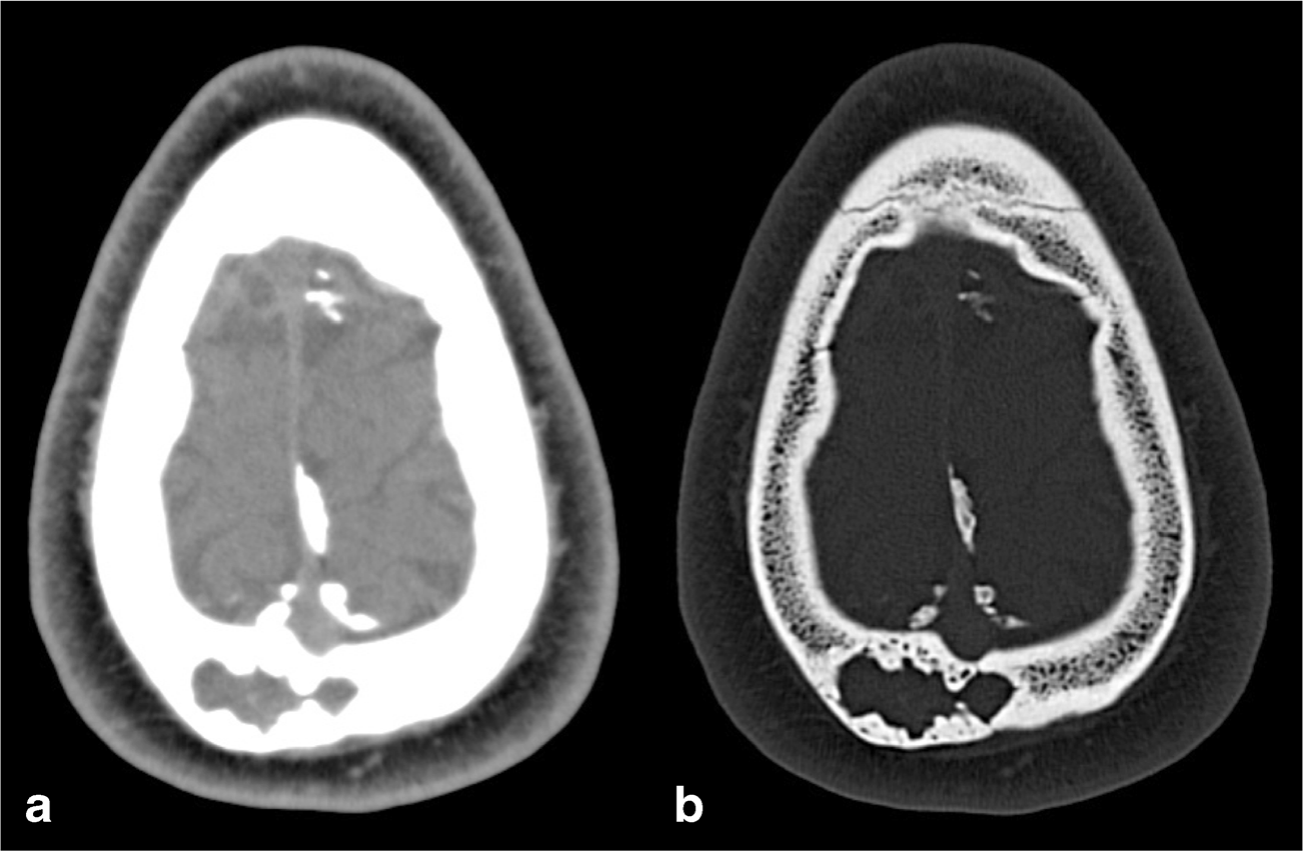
Bone desmoplastic fibroma (DF). Axial CT images with soft tissues (a) and bone (b) windows showing a right parietal lytic lesion without erosion of the inner and outer tables
